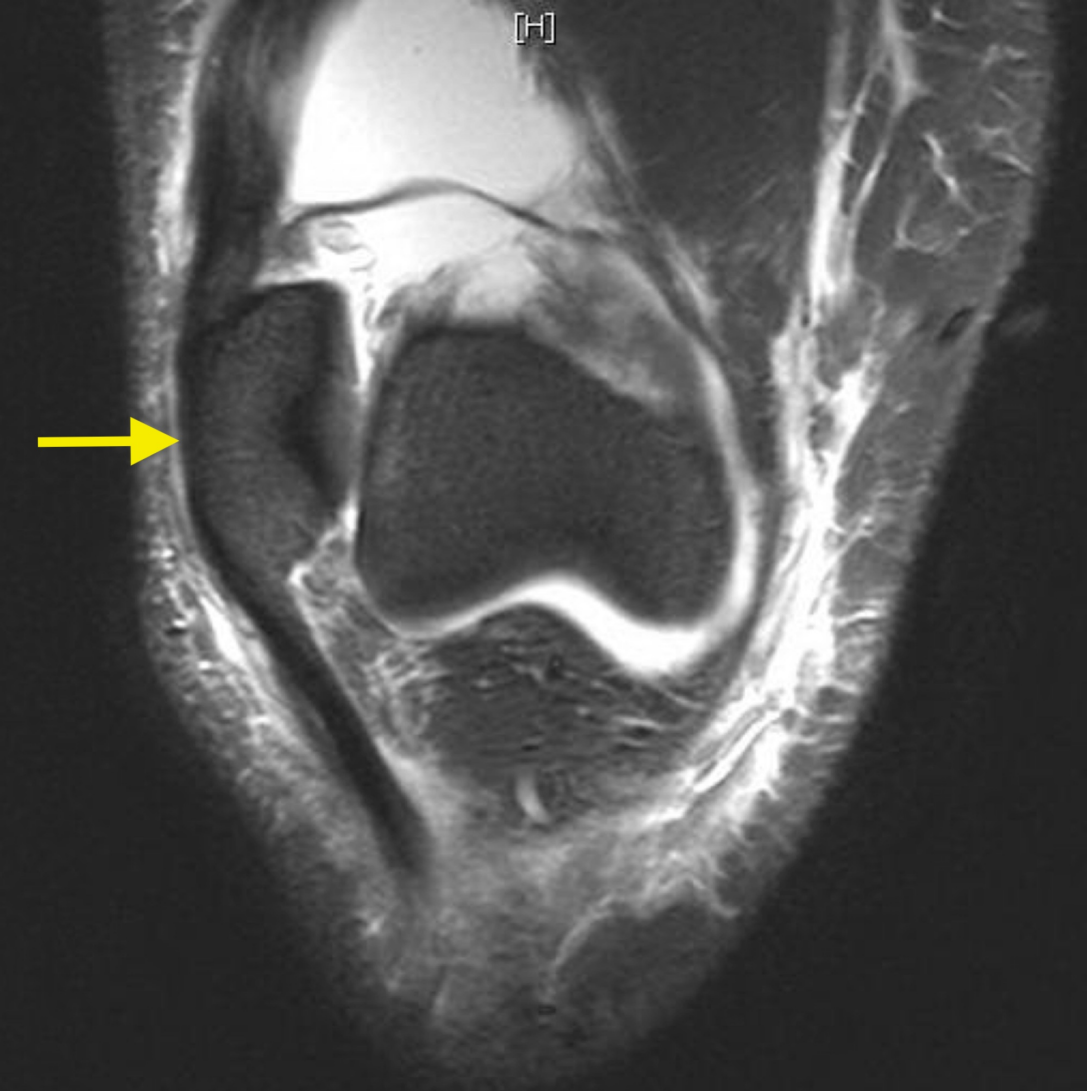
Lateralised, dislocated patella (yellow arrow) on coronal magnetic resonance image of right knee.
